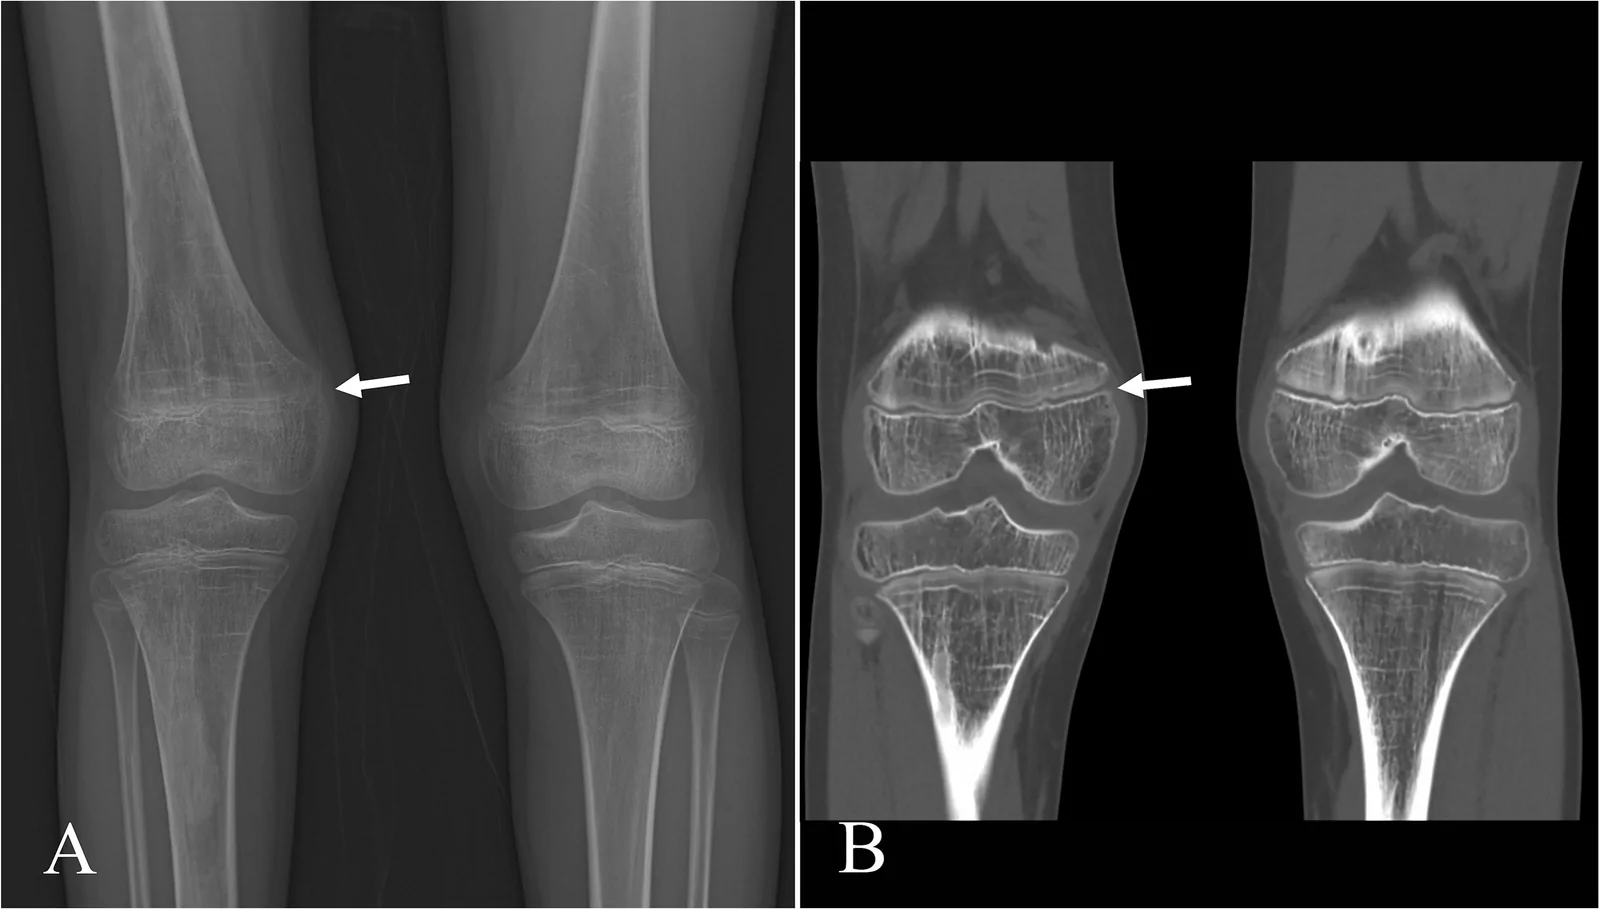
Follow-up imaging of Case 2 six months after burosumab initiation. Anteroposterior radiograph of both knees (A) and coronal CT images (B) show marked normalization of the previously widened and irregular distal femoral physes, with smoother physeal contours and resolution of adjacent metaphyseal fraying. Arrows indicate the medial distal femoral physis.
